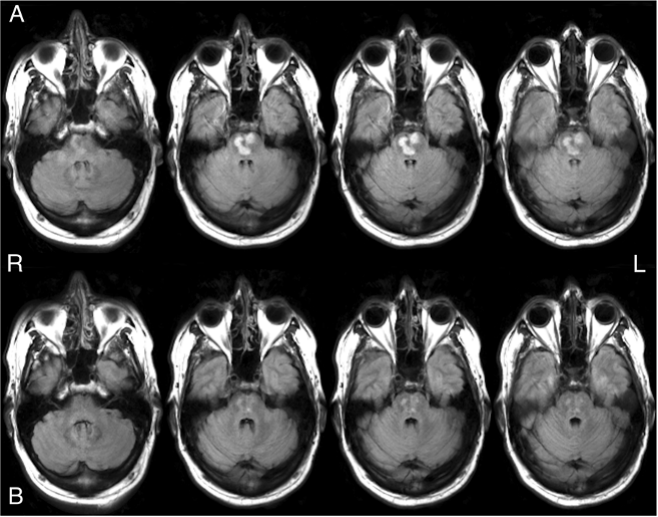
Baseline and follow-up fluid attenuated inversion recovery structural images. (A) Axial fluid attenuated inversion recovery images of the pontine infarct from the baseline magnetic resonance imaging scan. (B) Axial fluid attenuated inversion recovery images of the pontine infarct from the follow-up magnetic resonance imaging scan.
